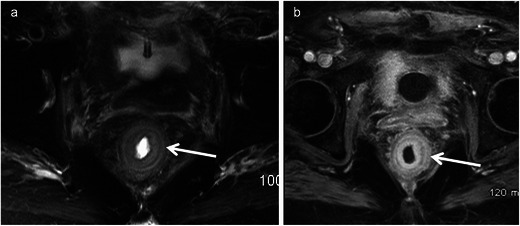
Secondary linitis plastica of rectum from breast cancer. a Axial T2-weighted MRI reveals concentric thickening of the rectal wall with a concentric ring pattern likely due to exaggeration of the zonal anatomy. b Post-contrast T1-weighted MRI demonstrates similar findings
